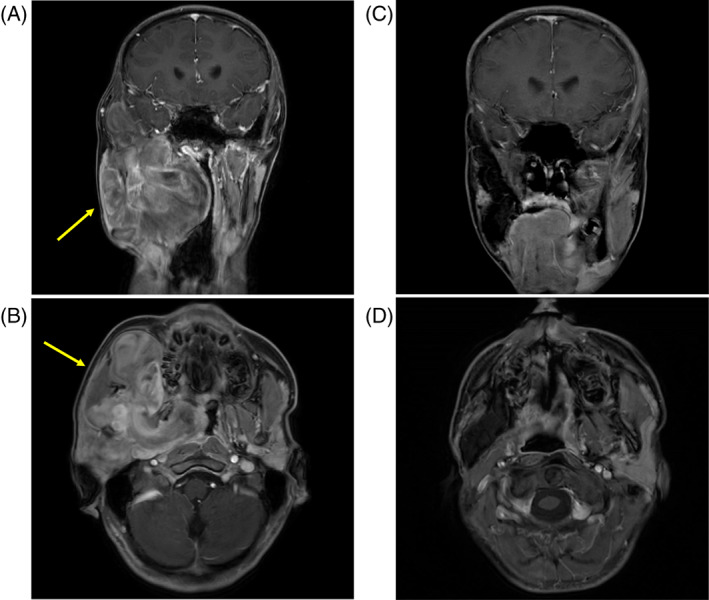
Contrast‐enhanced magnetic resonance imaging (MRI): (A and B) At diagnosis, the lobulated mass occupies the right side of the masticator space. The inside of the mass shows a non‐uniform contrast effect (Yellow arrow). (C and D) 3 years after completion of the therapy, no sign of recurrence is found.
